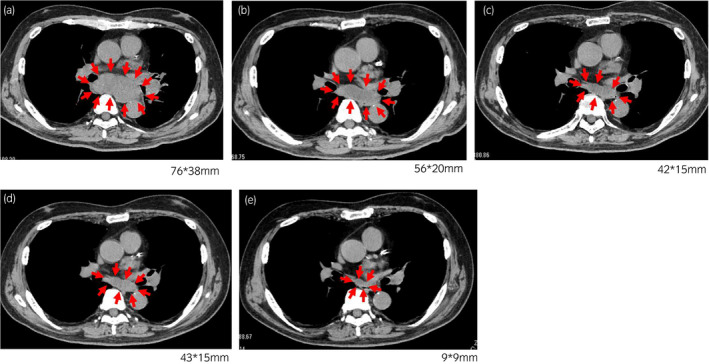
Computed tomography scan images demonstrate a decrease in size of mediastinum lymph nodes and lung metastases after three cycles of infusions of nivolumab. The size of mediastinum lymph node metastasis is shown in each figure. (a) Before treatment with nivolumab. (b) Just after three cycles of nivolumab administration. (c) Six months after last nivolumab administration. (d) One year after last nivolumab administration. (e) Two years after last nivolumab administration.
